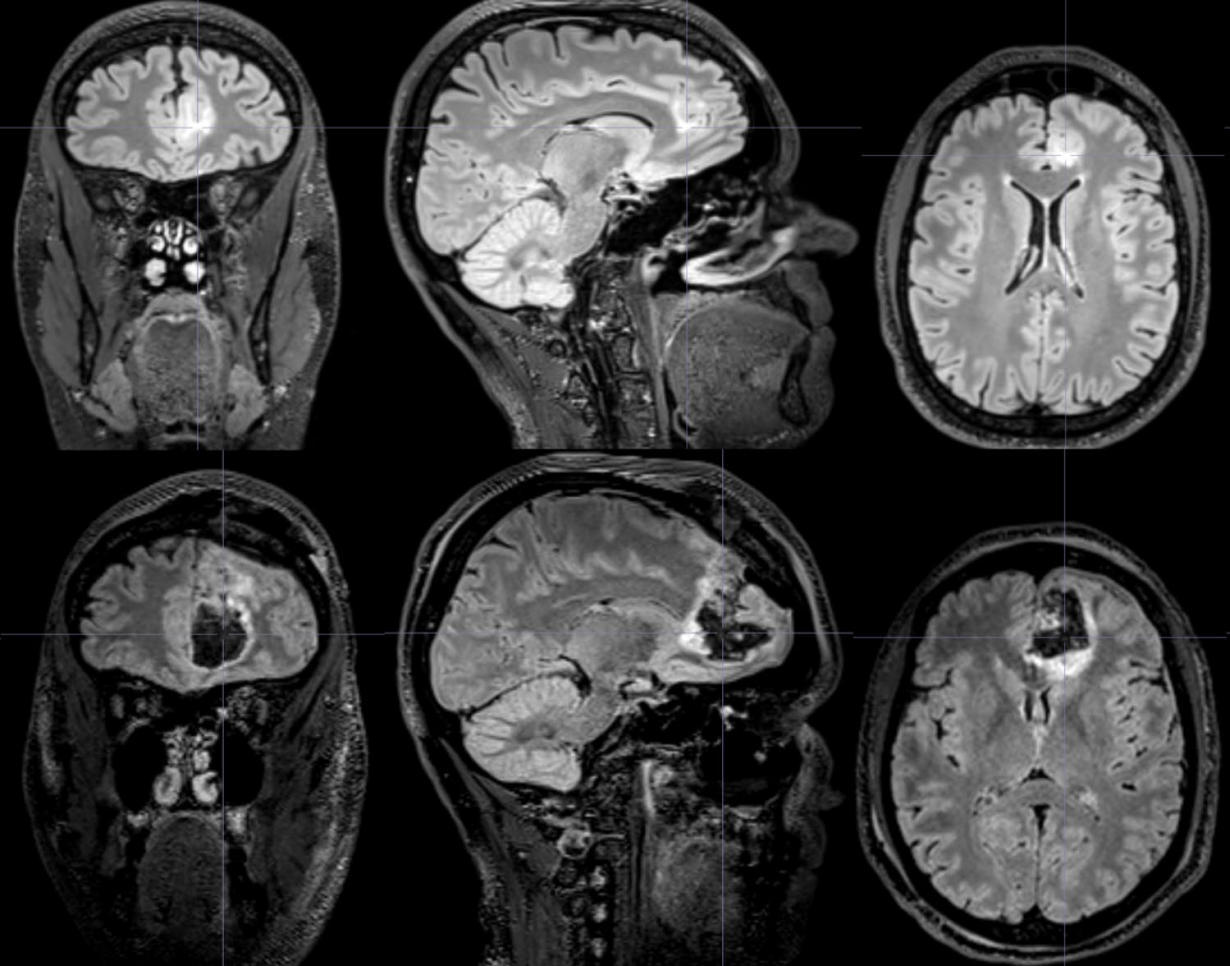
Pre- and postoperative imaging. (Upper row) Coronal, sagittal, and axial T2-FLAIR MRI slices showing a hyperintense lesion in the left anterior cingulate cortex. (Lower row) Postoperative T2-FLAIR images confirming the extended lesionectomy
